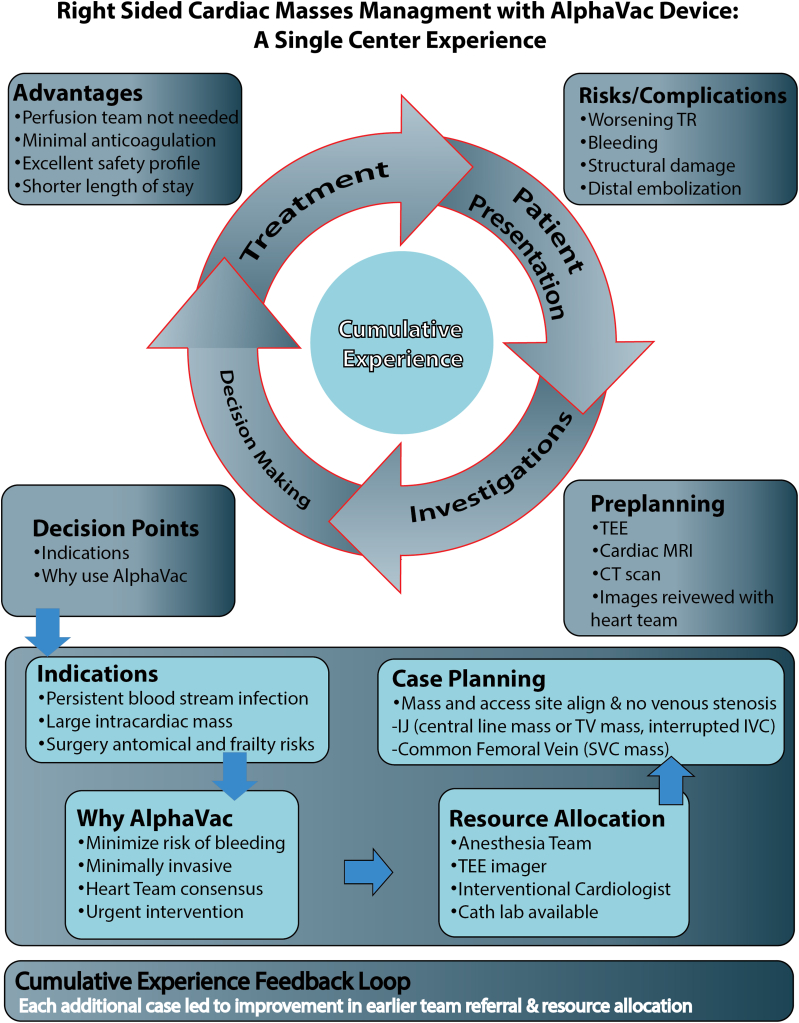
Right sided cardiac masses management with AlphaVac device: a single center experience. CT, computed tomography; IJ, internal jugular; IVC, inferior vena cava; MRI, magnetic resonance imaging; SVC, superior vena cava; TEE, transesophageal echocardiogram; TR, tricuspid regurgitation; TV, tricuspid valve.
